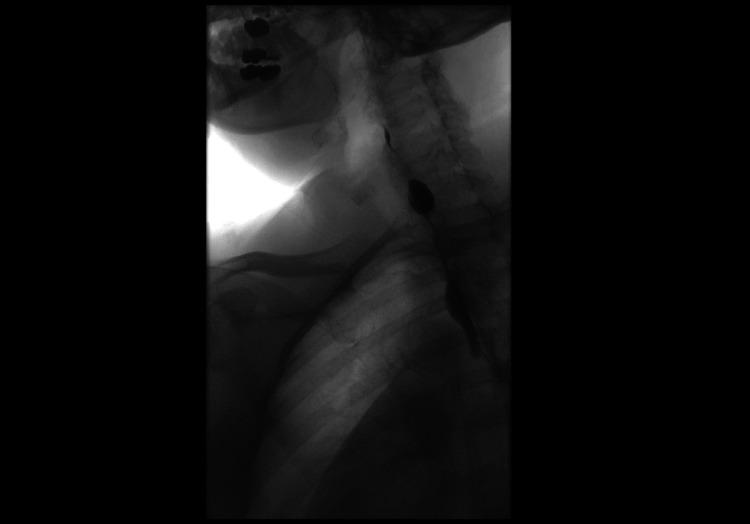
Barium swallow X-ray image taken one month following the patient's hospital stay. Image shows evidence of contrast pooling proximally to a focal esophageal stricture.
